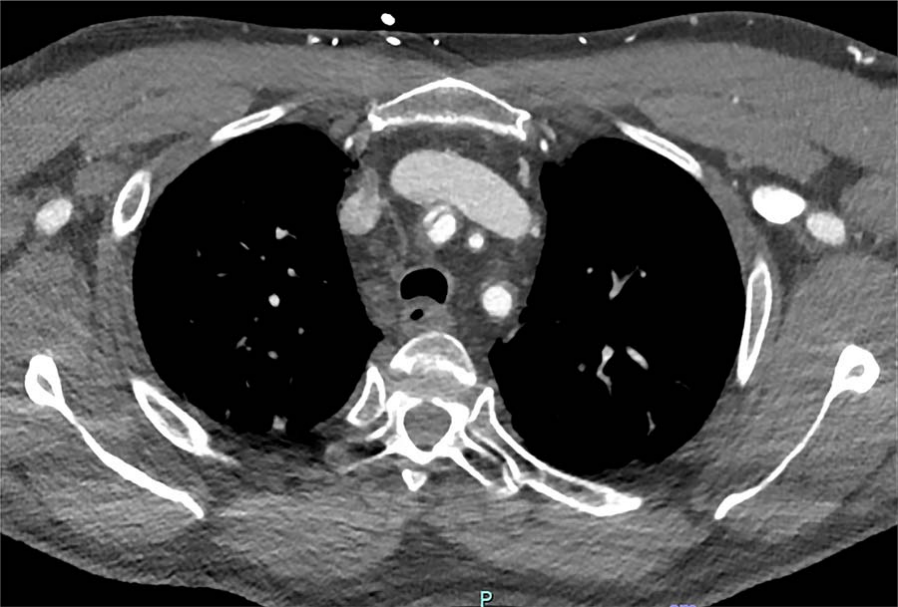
Axial slice from CT aortogram demonstrating the proximal aspect of the dissection flap.
